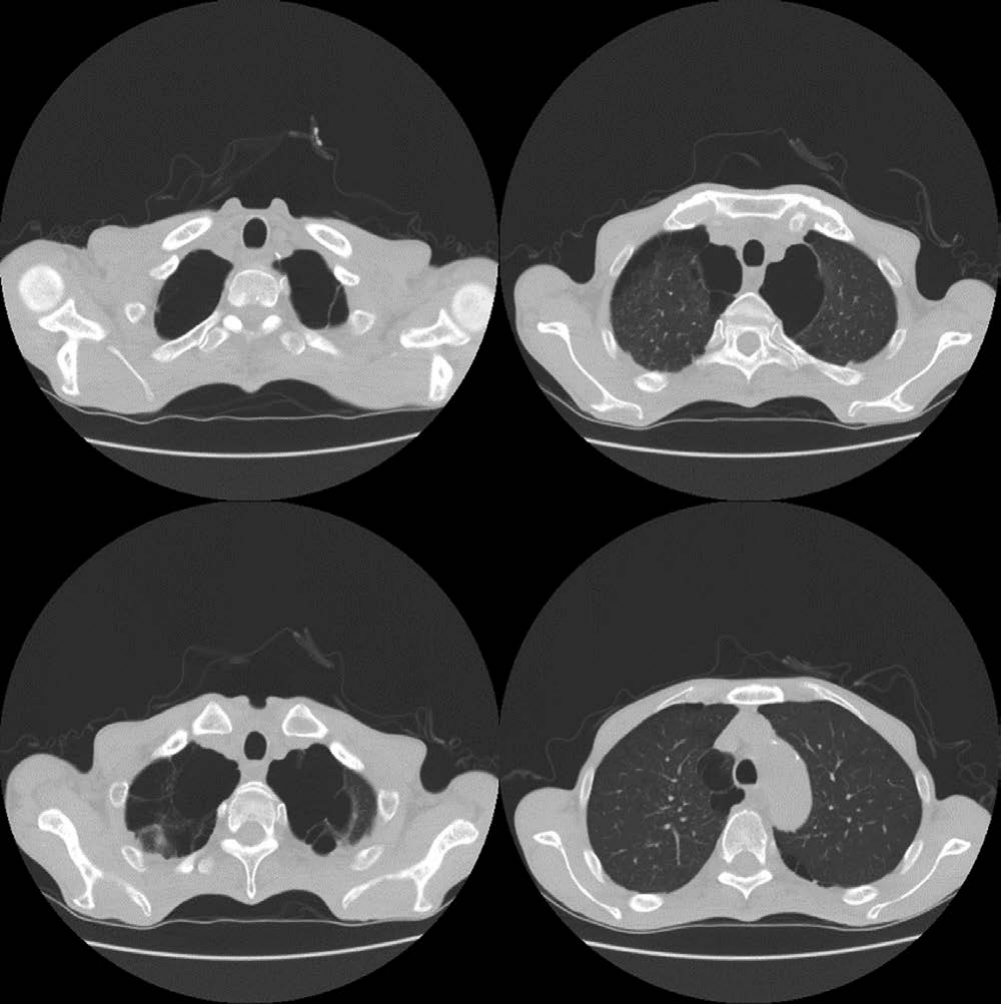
Preoperative chest computed tomography shows severe emphysematous lungs.
